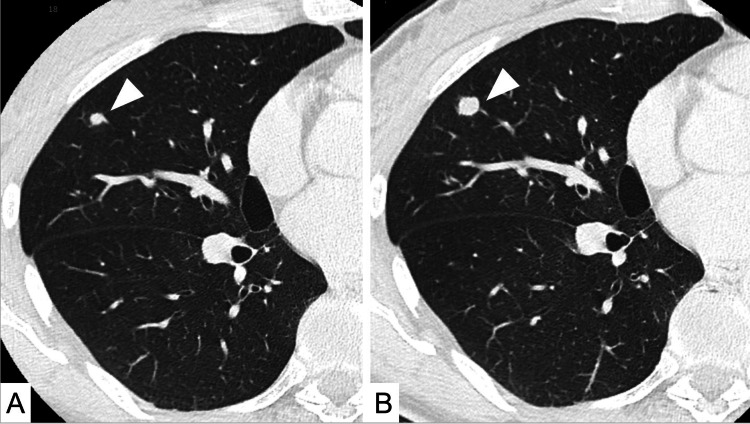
Chest CT findings. (A) Plain axial CT image showing a 5.8 mm nodule in the right middle lobe (arrowhead). (B) The nodule enlarged to 9 mm over four years (arrowhead).
